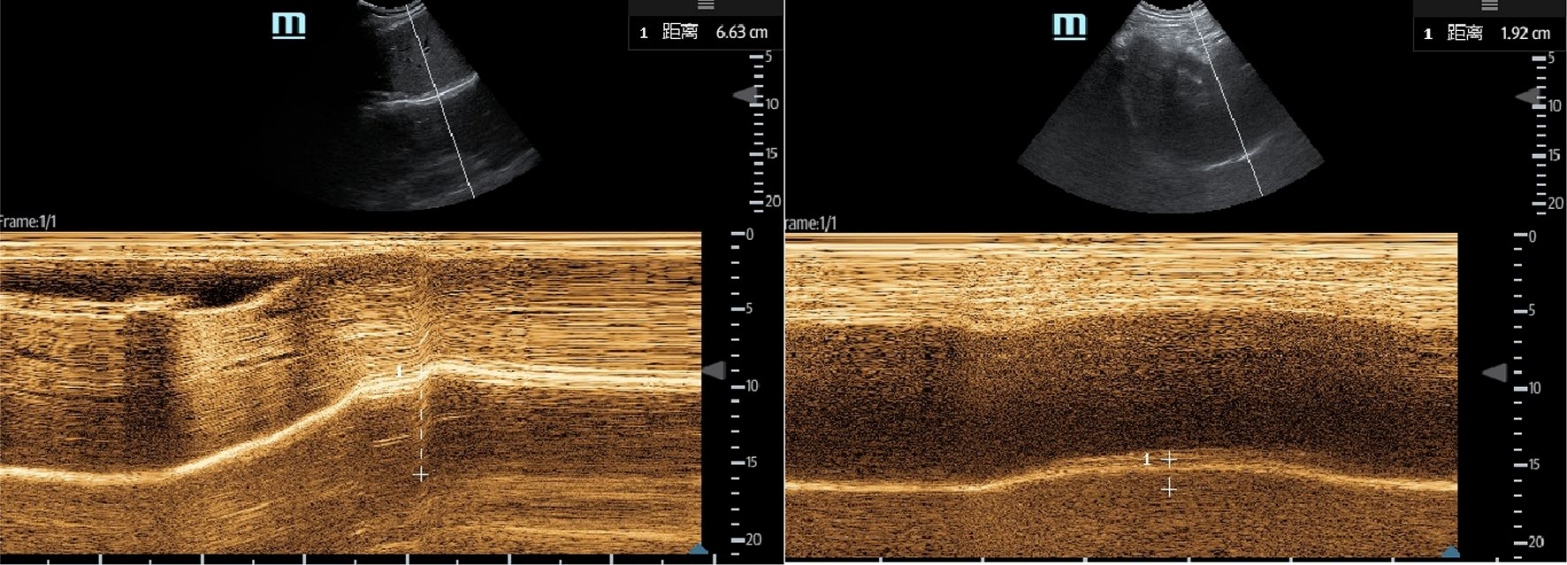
Real-time M-mode ultrasonography of the hemidiaphragm
